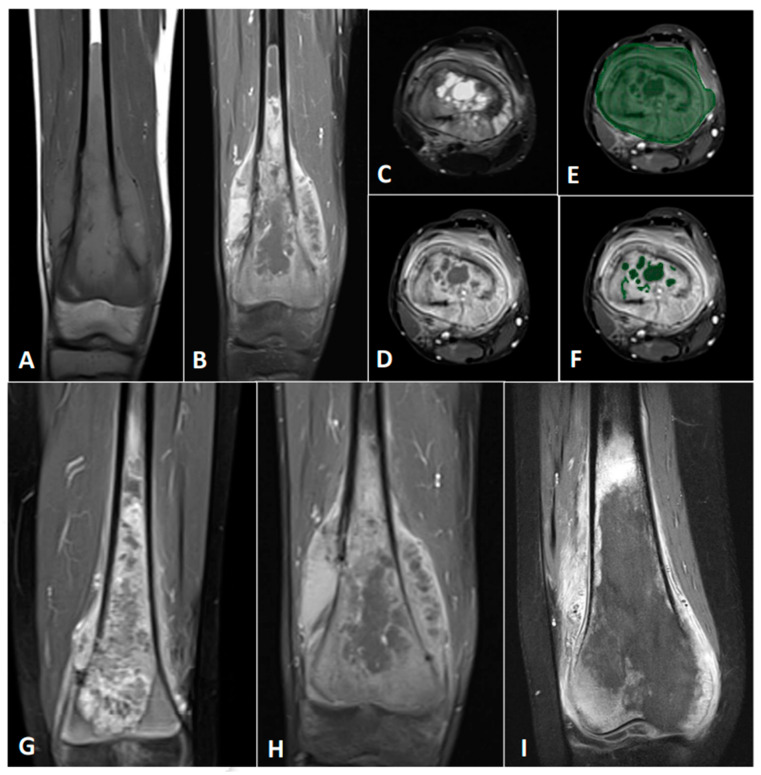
Magnetic resonance imaging (MRI) analyses. (A–D). Example of a high-grade osteosarcoma (OTS) at diagnosis in a 15-year-old patient: coronal T1 weighted image (A), post-contrast fat-saturated (FS) T1 weighted image (B), axial FS T2 weighted image (C) and post-contrast axial FS T1 weighted image. (E) Manual delineation of the tumor volume margins. (F) Manual delineation of intra-tumor necrotic areas. (G–I) Three different levels of necrosis in bottom line figures: coronal post-contrast FST1 weighted MRI images with a small proportion of necrosis (G), an average proportion of necrosis (H) and a high percentage of necrosis (I).
